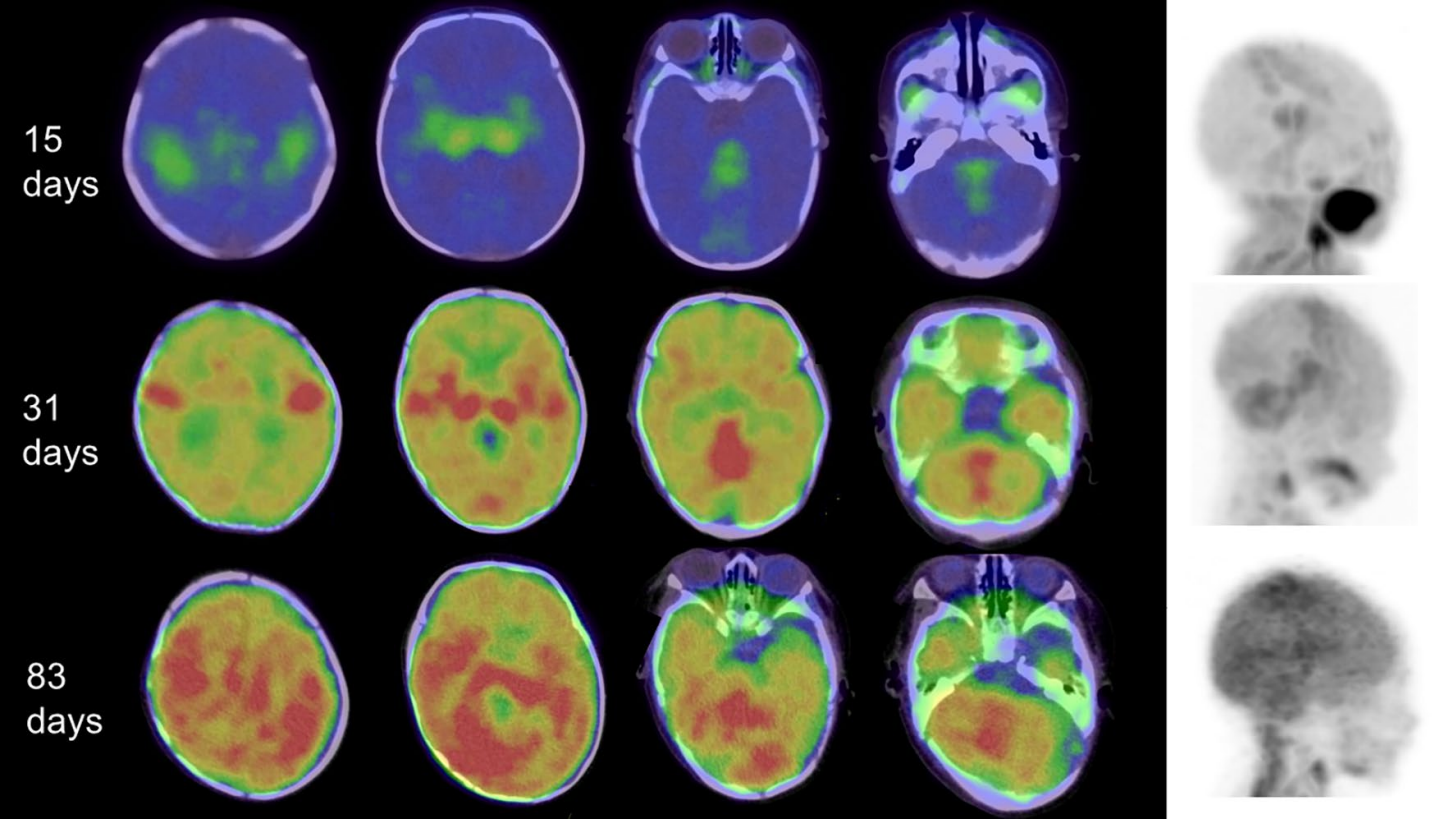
Alternation of brain FDG uptake from neonate to infant. In neonate (15 days after birth), FDG uptake for sensorimotor cortex (left end), thalamus (2^nd^ from the left), brain stem (3^rd^ from the left), cerebellar vermis (right end) are prominent. In infant with one month after the birth, slight increase in FDG uptake in the brain hemispheres. FDG uptake in the brain hemispheres becomes more prominent after 2 months from birth. In addition, CT imaging of neonates and infants reveals that the cranial bones are not yet fully fused, showing gaps at the sutures
